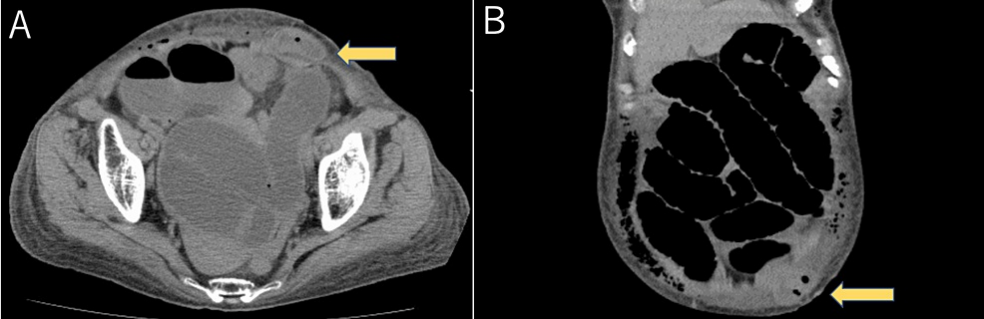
Postoperative axial (A) and coronal (B) CT images in Case 1 The images showed the small intestinal incarceration through an anterior abdominal wall (yellow arrow) with surrounding subcutaneous edema.
